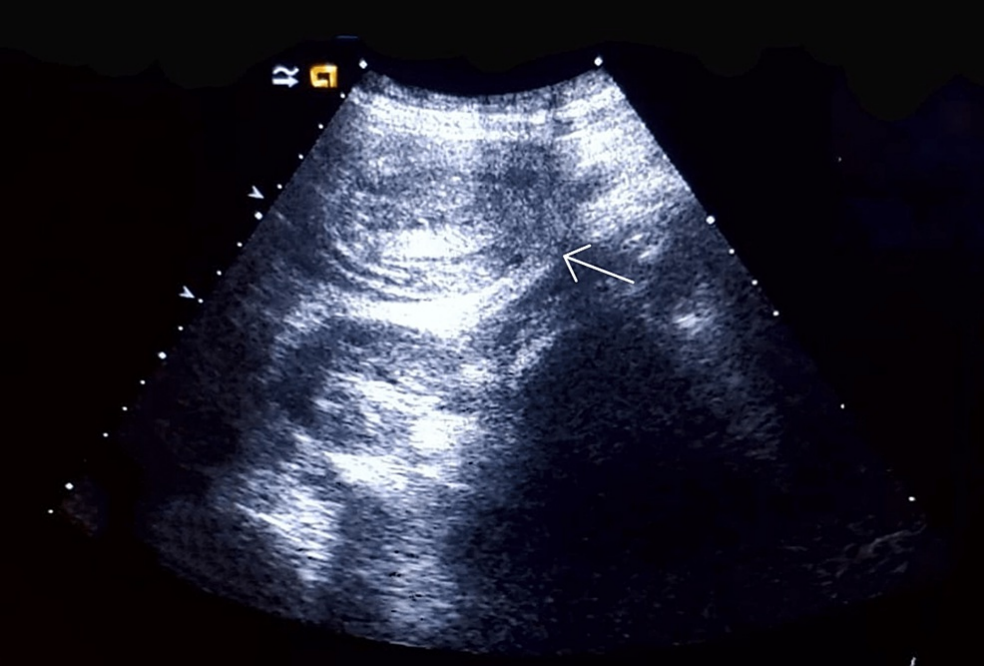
Long-axis 1-4 MHz ultrasound image obtained over the right lumbar region demonstrates the pseudokidney sign (long-axis diameter of 6.50 cm)
